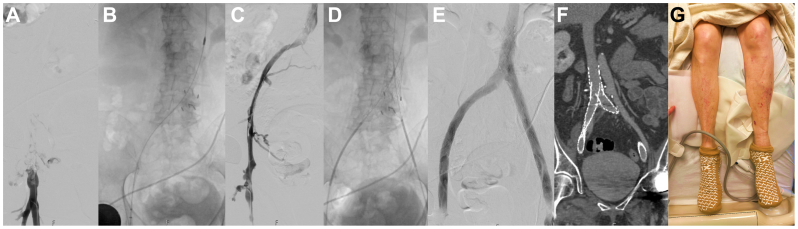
Attention was then turned to endovascular mechanical thrombectomy for deep vein thrombosis (DVT). (A) A venogram demonstrated occlusive thrombus in the left common femoral, external iliac, and common iliac veins. (B) Mechanical thrombectomy was pursued with a ClotTriever Bold catheter. (C) After thrombectomy, there was re-establishment of flow in the iliac veins, albeit with chronic-appearing long-segment stenosis and webs. (D) Iliocaval reconstruction was pursued. In the inferior vena cava (IVC), a 20 × 6 cm Abre stent was placed. Kissing 14 mm × 15 cm (left iliac) and 14 mm × 12 cm (right iliac) Abre stents were placed. (E) Completion venogram showed widely patent iliocaval reconstruction. (F) Postoperative computed tomography (CT) venogram demonstrated patent iliocaval reconstruction, with a single slice focusing on the right iliac reconstruction. (G) Patient legs at 24 hours after intervention showing significant improvement in swelling, size, and purpura of the left lower extremity, with near normal appearance.
